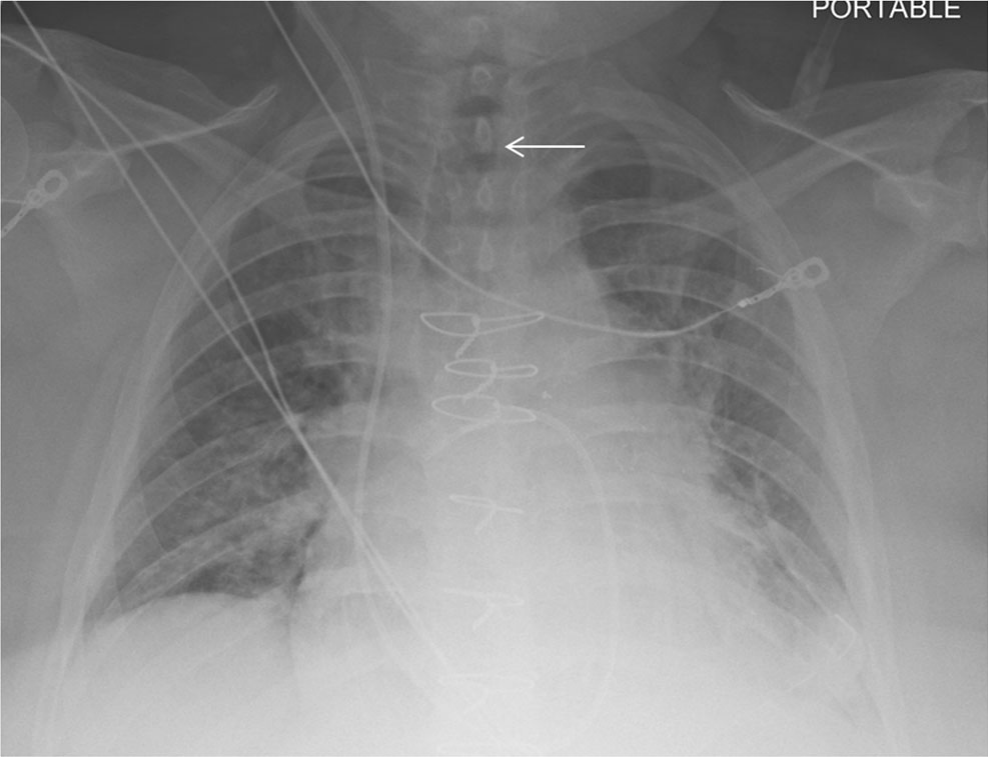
Follow-up chest radiograph on the same patient after ET tube has been removed. The *white arrow* depicts the absence of the ET tube
